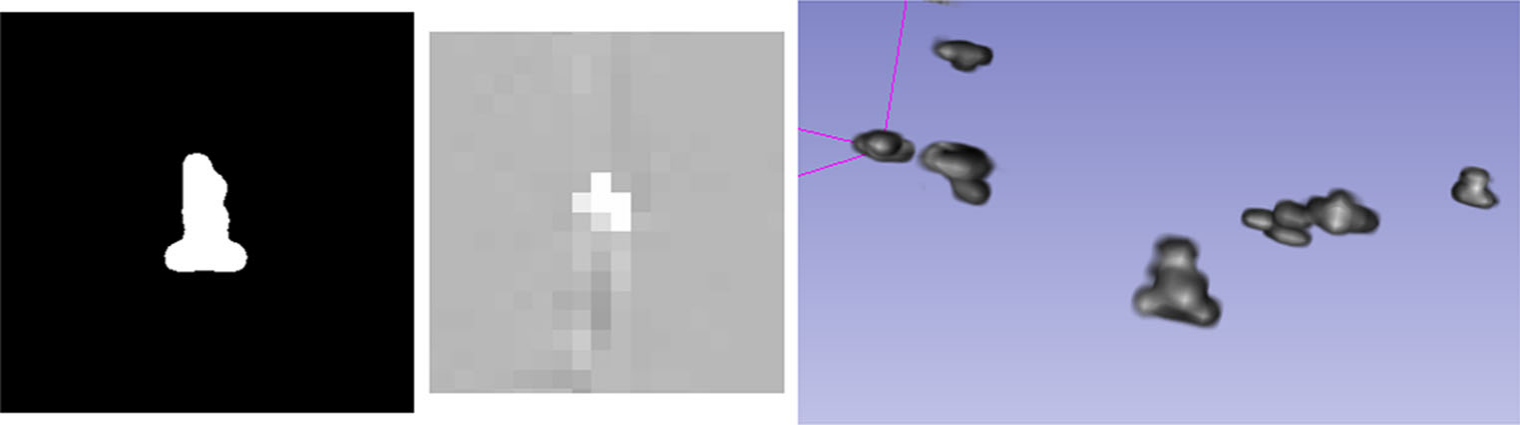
The image on the *left side* shows a cropped sample of the virtual CT image around a screw, after choosing an appropriate Hounsfield intensity window. The *middle image* shows a view of a screw without interpolation and with a different window selection. The image on the *right side* shows a volume-rendered screenshot of the segmented virtual scene within Slicer
